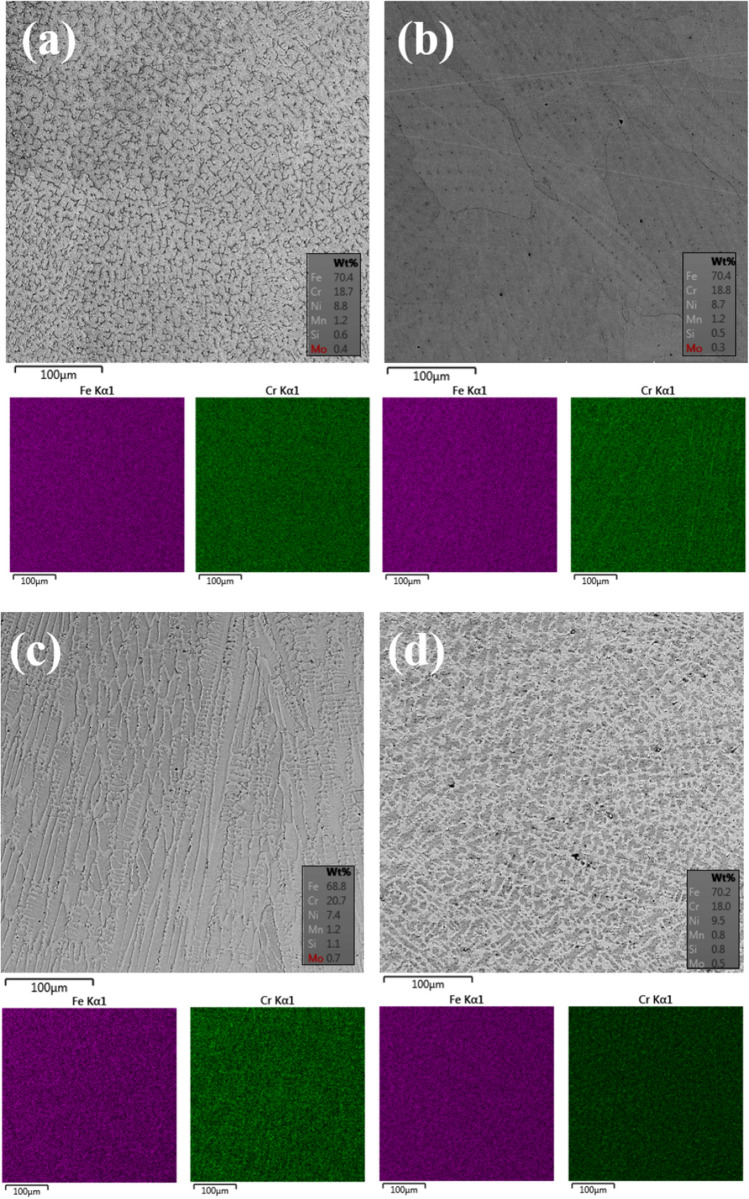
Microstructure of the metal matrix to (a) 304LSS, 304LSS-CNT with (b) MA, (c) CT_TT_, and (d) CT conditions.
